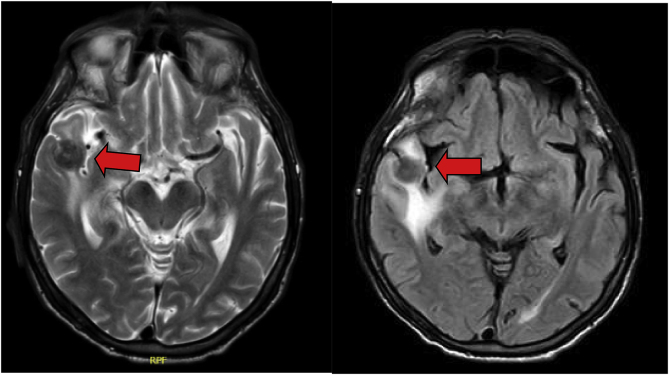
(Case 1) Day +2 Brain MRI (T2 and FLAIR). Multiple focal cortical and subcortical lesions diffusely distributed in the supratentorial and infratentorial regions, and the left pontine (red arrows). (For interpretation of the references to colour in this figure legend, the reader is referred to the Web version of this article.)
